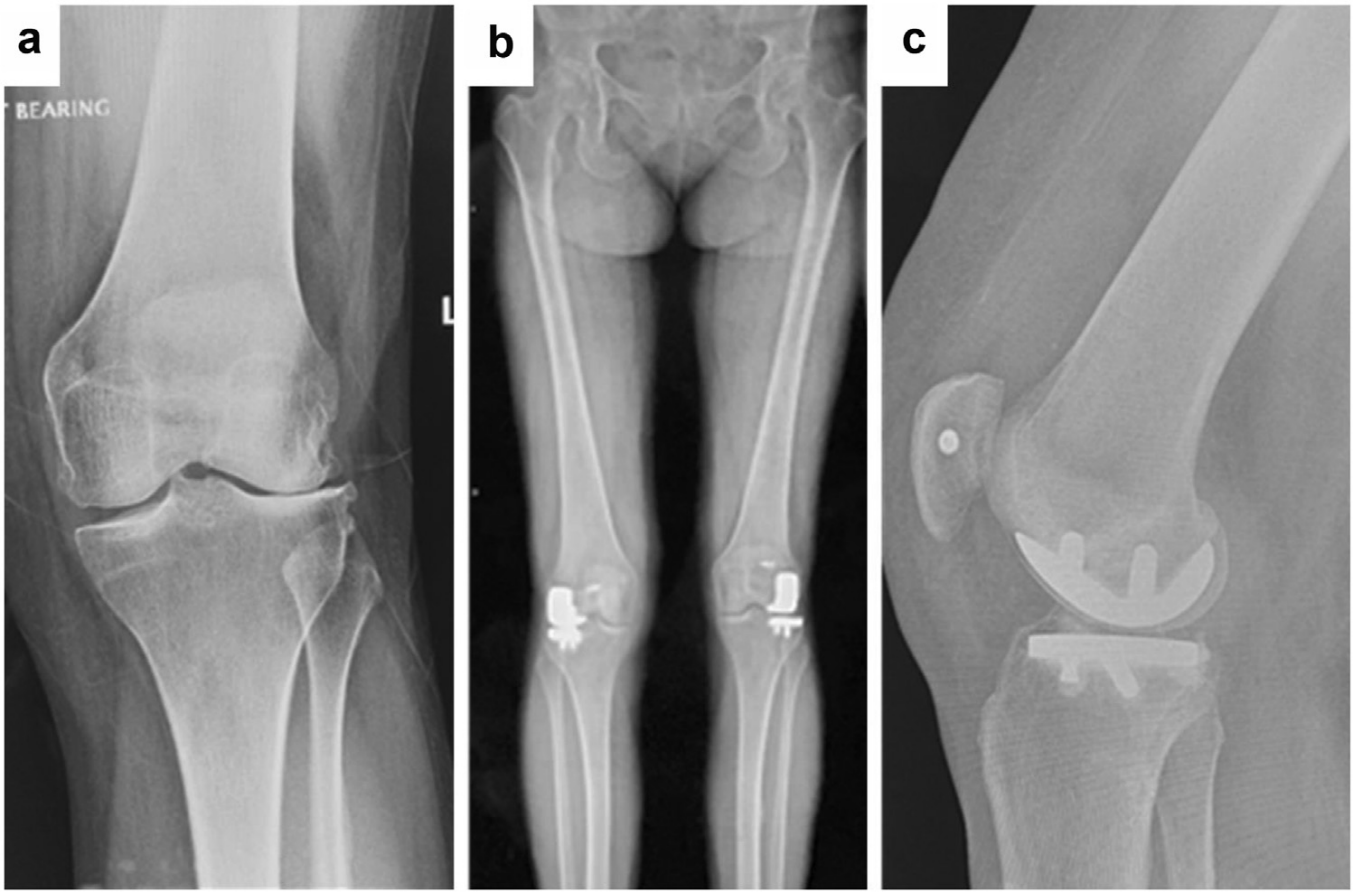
Pre- and post-operative radiograph of patient with isolated lateral unicompartmental OA who underwent a lateral UKA using the Triathlon PKR implant. (a) Preoperative anterior-posterior radiograph, (b) postoperative anterior-posterior radiograph, (c) postoperative lateral radiograph.
